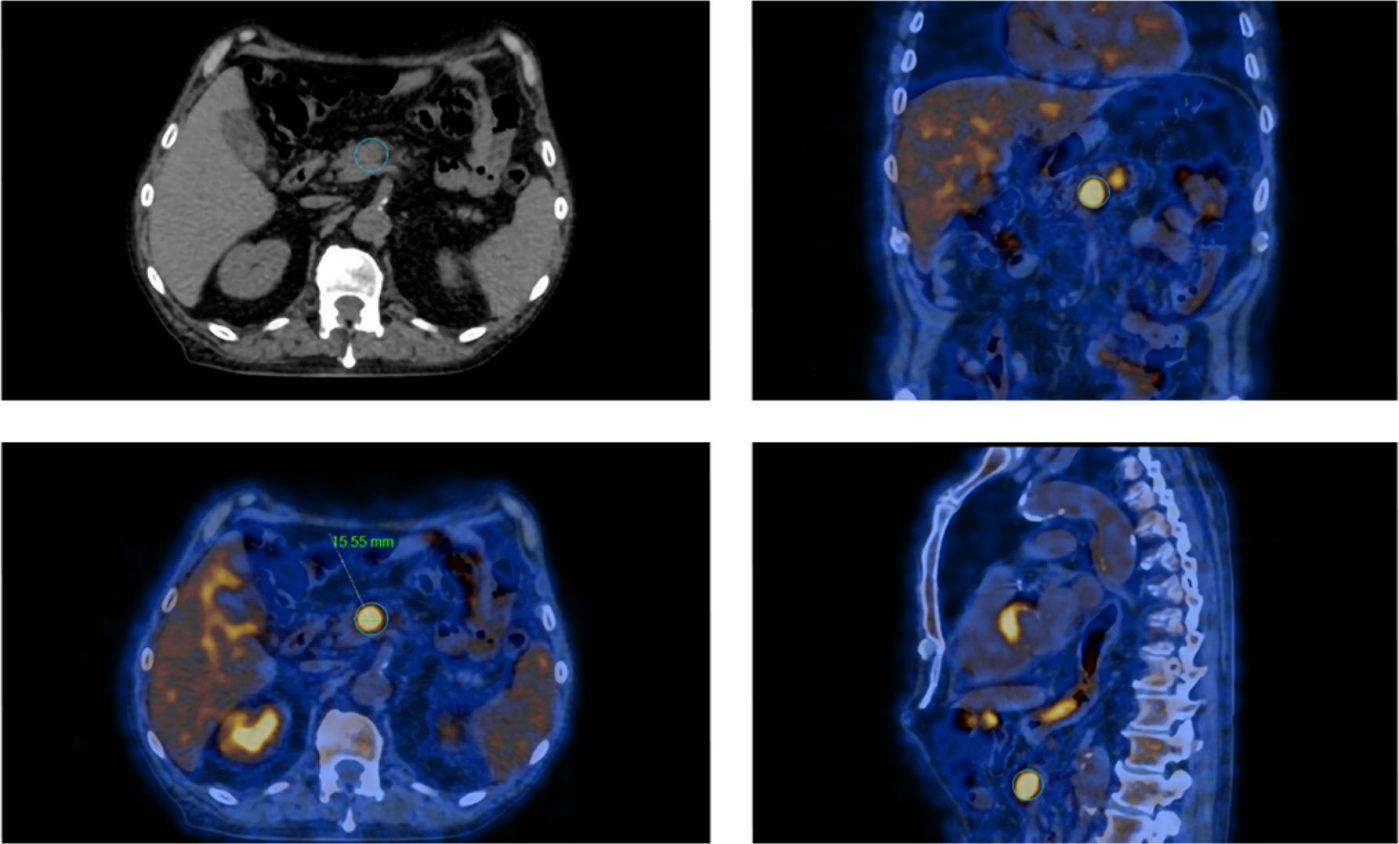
Case 1, 18-FDG–PET/CT scans of pancreatic and lymph node hypermetabolism. *18-FDG* 18 fluorodeoxyglucose, *PET/CT* positron emitting tomography/computed tomography
